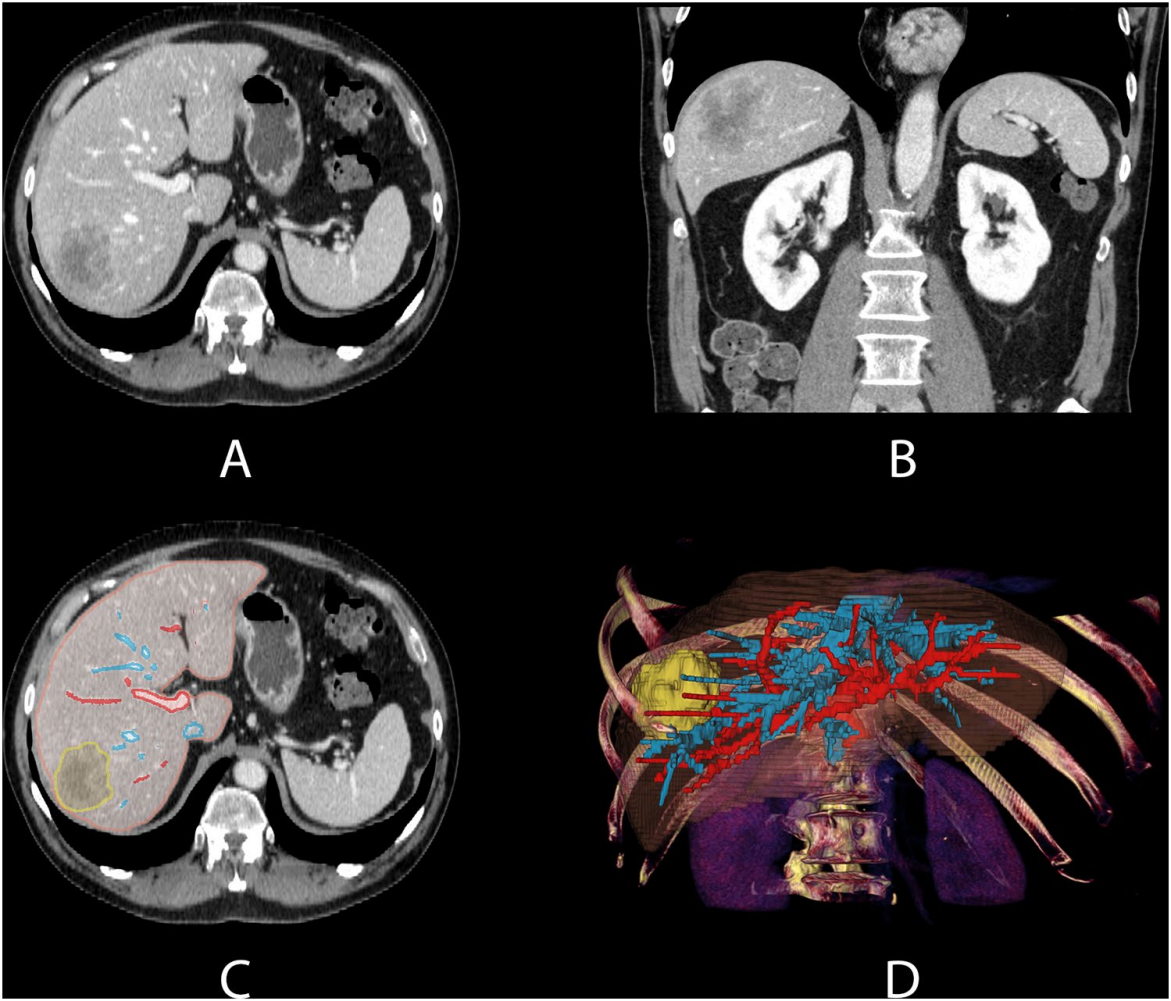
Patient-specific 3D model based on preoperative CT scan
